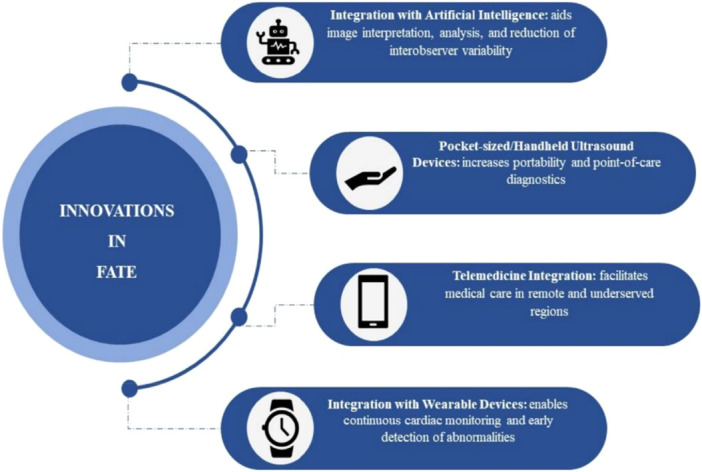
Emerging technologies and innovations in Focused Assessment Transthoracic Echocardiography (FATE).
